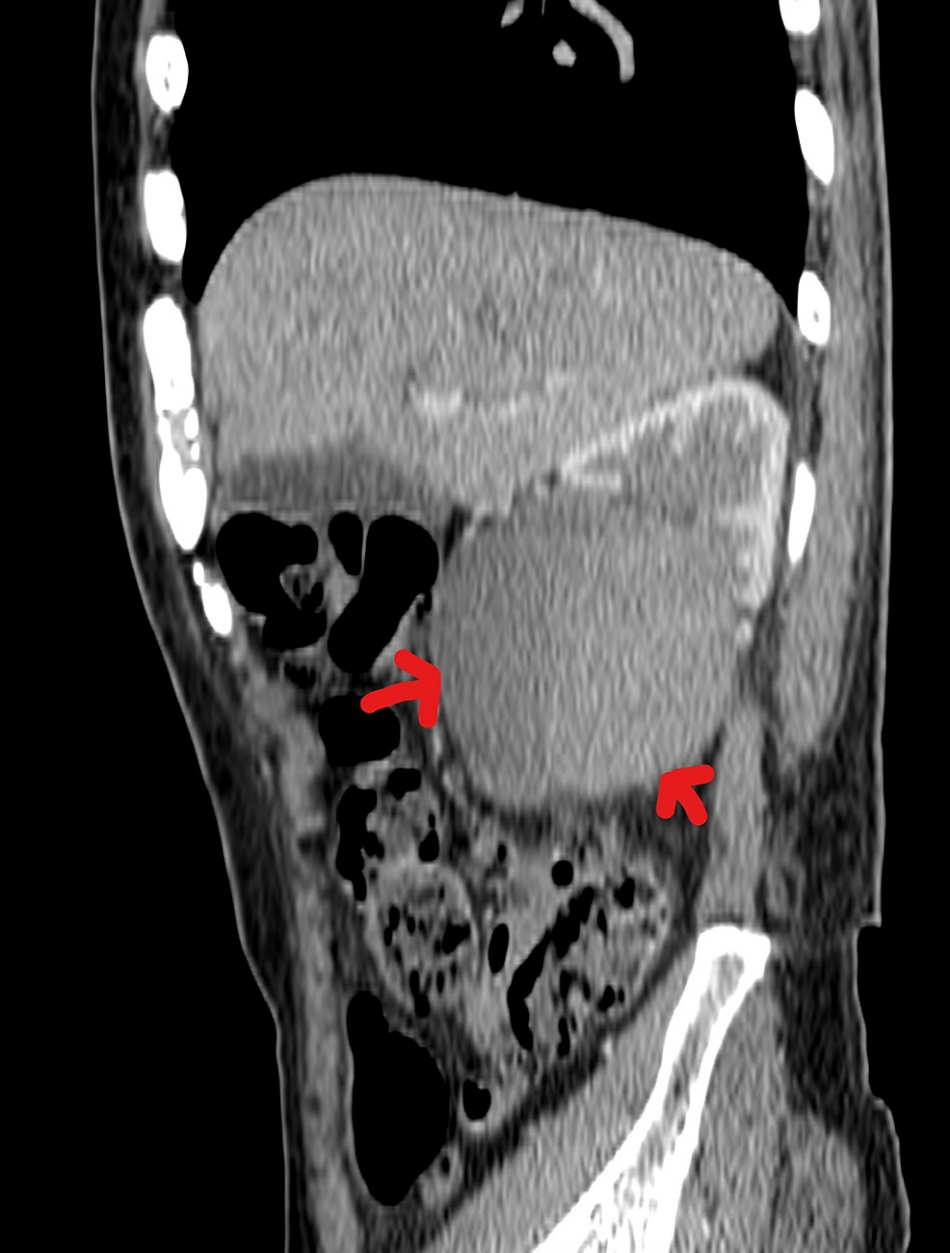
Non-contrast CT scan (sagittal view) through the level of the right kidney showing a large hematoma contained by a massively dilated renal pelvis (red arrows).
